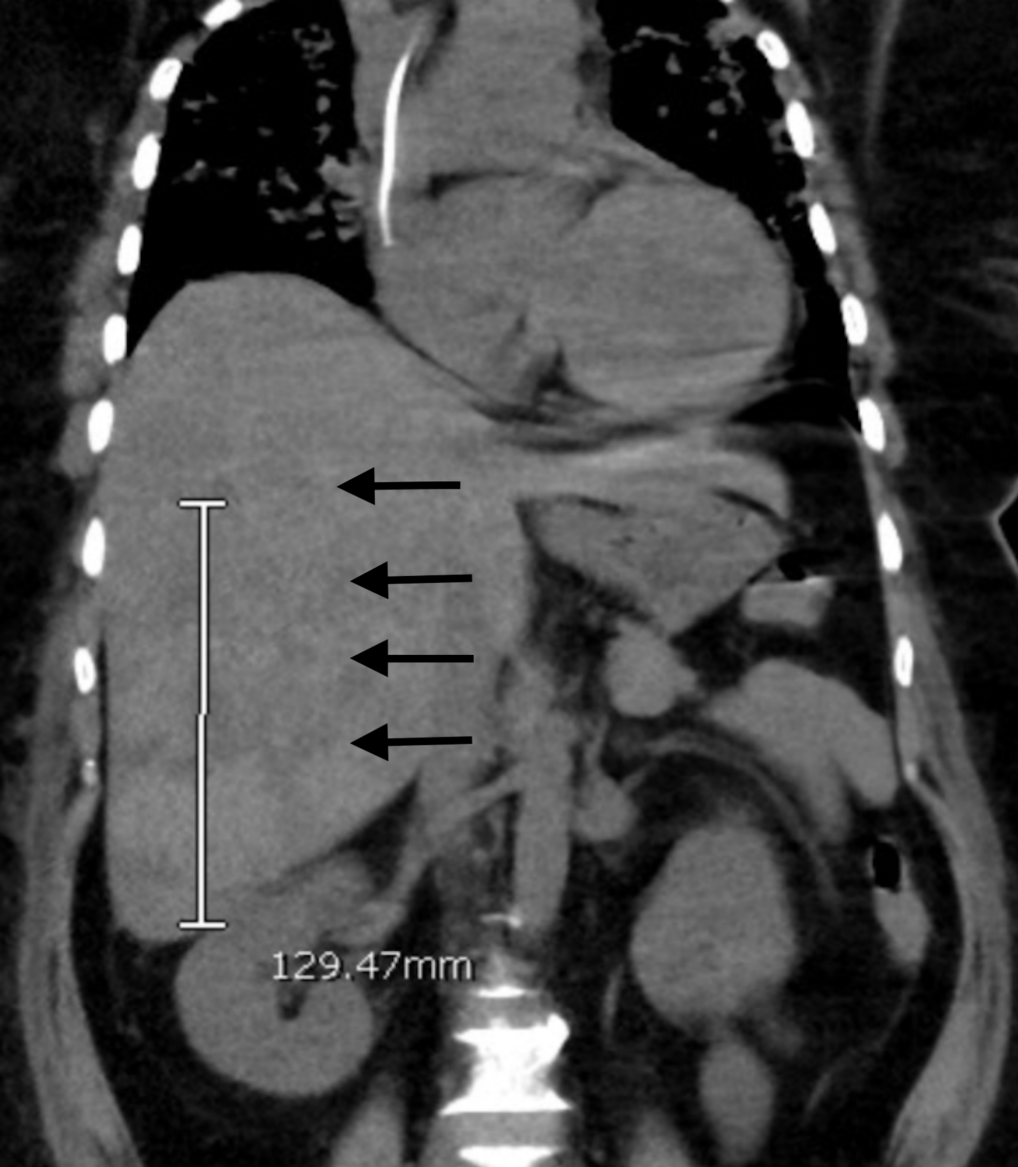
Non-contrast CT scan of the abdomen and pelvis showing heterogeneous area in the right side of the liver on the 15th day of hospitalization
